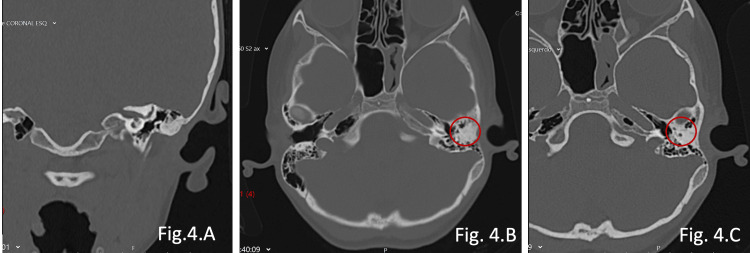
Temporal bone CT scan - coronal (A) and axial (B and C) views of Patient 2. A complete bony atresia of the external auditory canal (red circle), with a malformed middle ear on the left side, is seen. A very thin temporal bone is evident. The right ear anatomy is normal.
